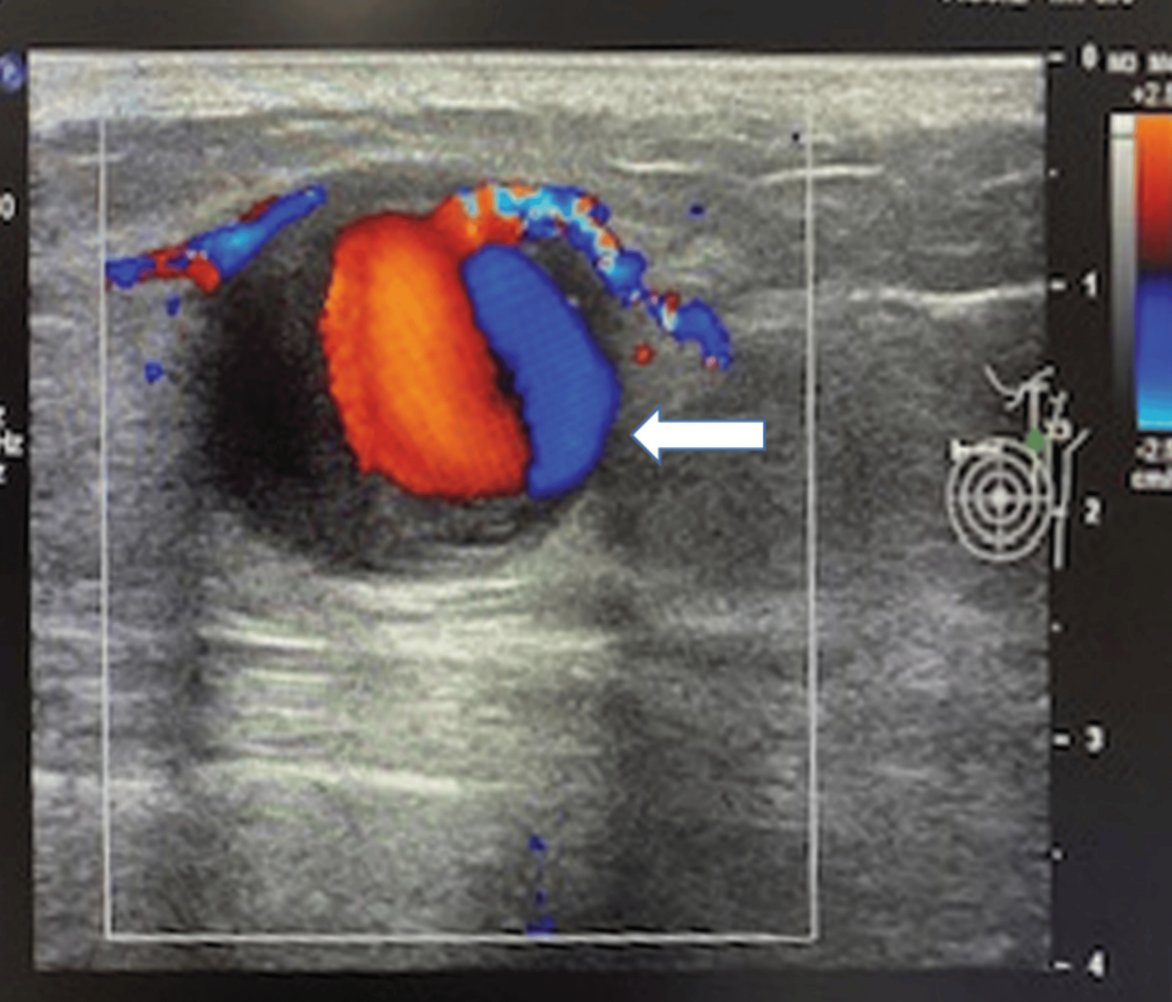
Doppler ultrasonography showing a pseudoaneurysm with a “yin-yan” flow pattern (white arrow) in the upper-inner quadrant of the left breast.
